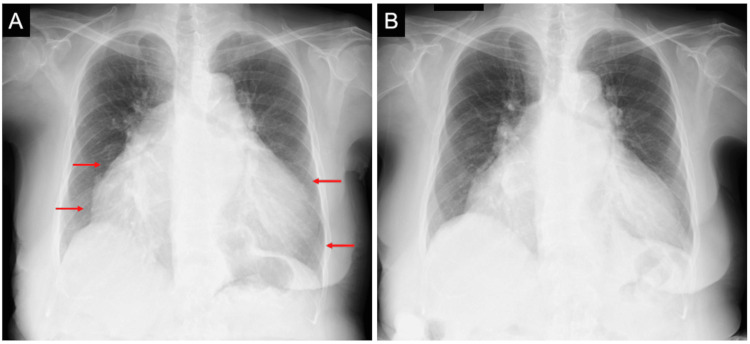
Initial and follow-up chest radiographs. Initial chest radiograph showed a significantly enlarged cardiac silhouette with a cardiothoracic ratio of 85%, mainly depending on right atrial and ventricular dilatations (A, arrows). The follow-up chest radiograph revealed a slight decrease in the cardiothoracic ratio to 80% (B).
